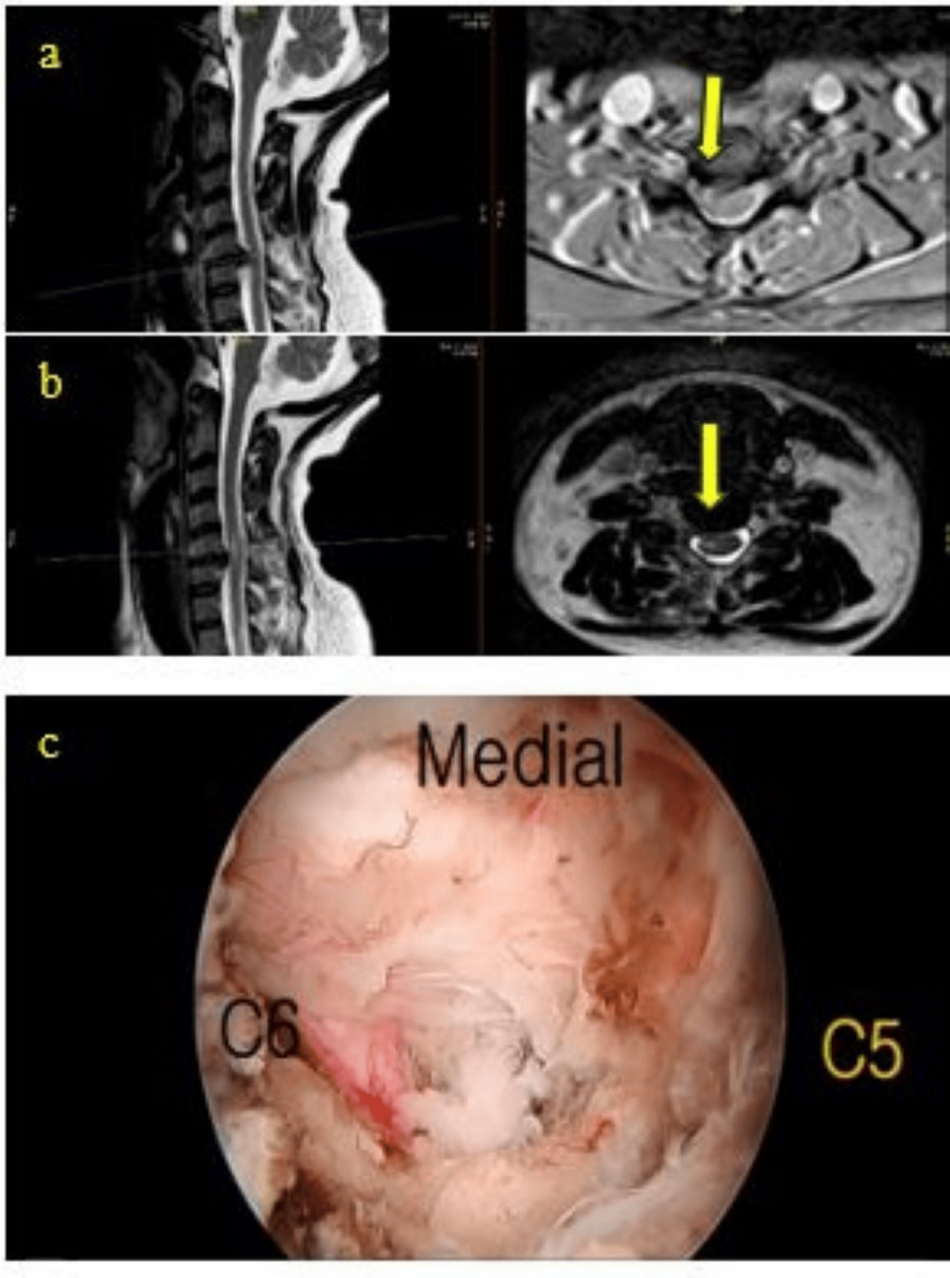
Unique Sensory Deficits (a) Preoperatively, axial and sagittal T2 MRI showing right C5/C6 disc herniation provoking the compression of the root and spinal cord (yellow arrow). (b) MRI in the axial and sagittal T2 sequences of the postoperative period showing the decompression of the nerve root and spinal cord with a hyperintense signal on the right anterolateral aspect (yellow arrow). (c) The intraoperative picture displays a cervical nerve root with an atypical cranial direction
